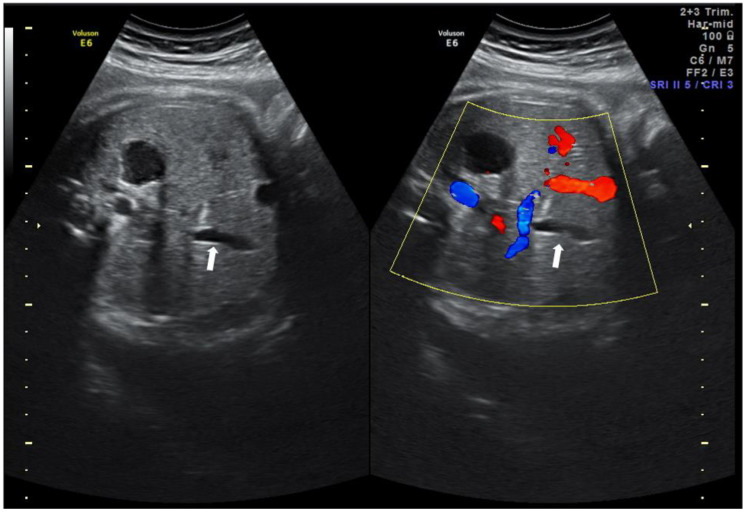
Image of the fetal gallbladder (white arrow) noted using 2D ultrasound with and without color.
